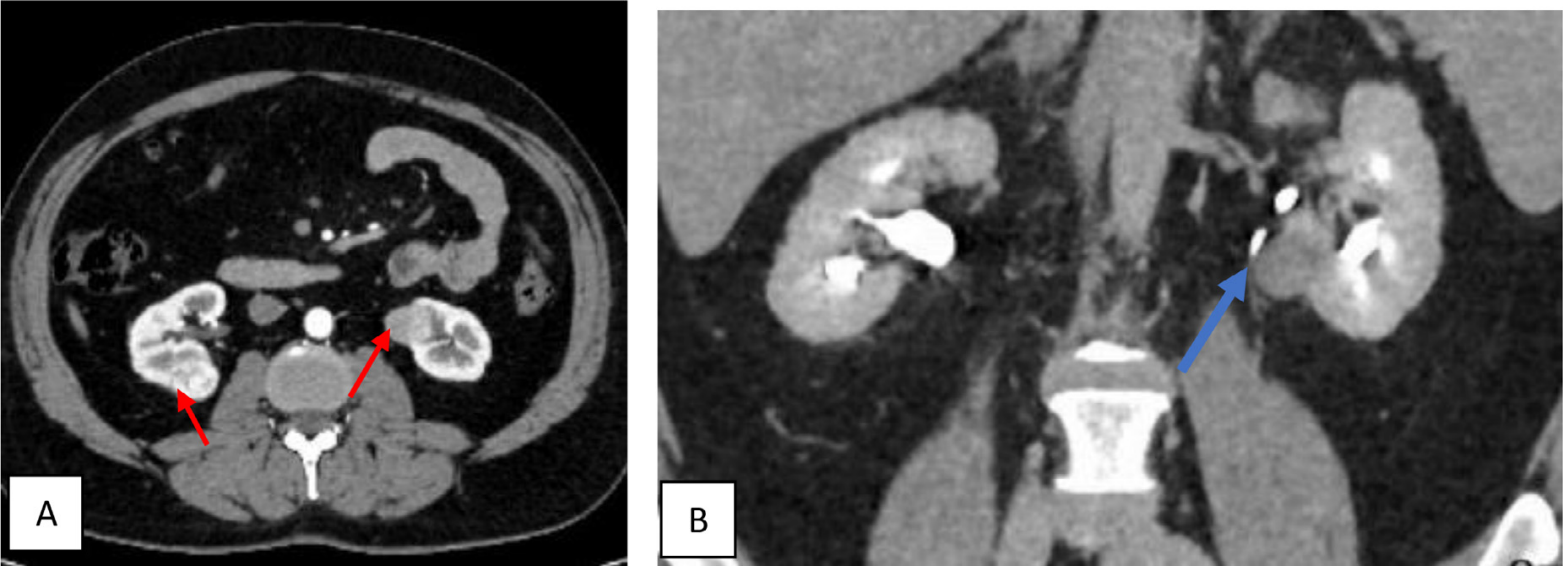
CT scan showing (A): bilateral small renal masses in axial section s (red arrows), (B) left renal mass in lower pole with proximity to left upper ureter (blue arrow).
